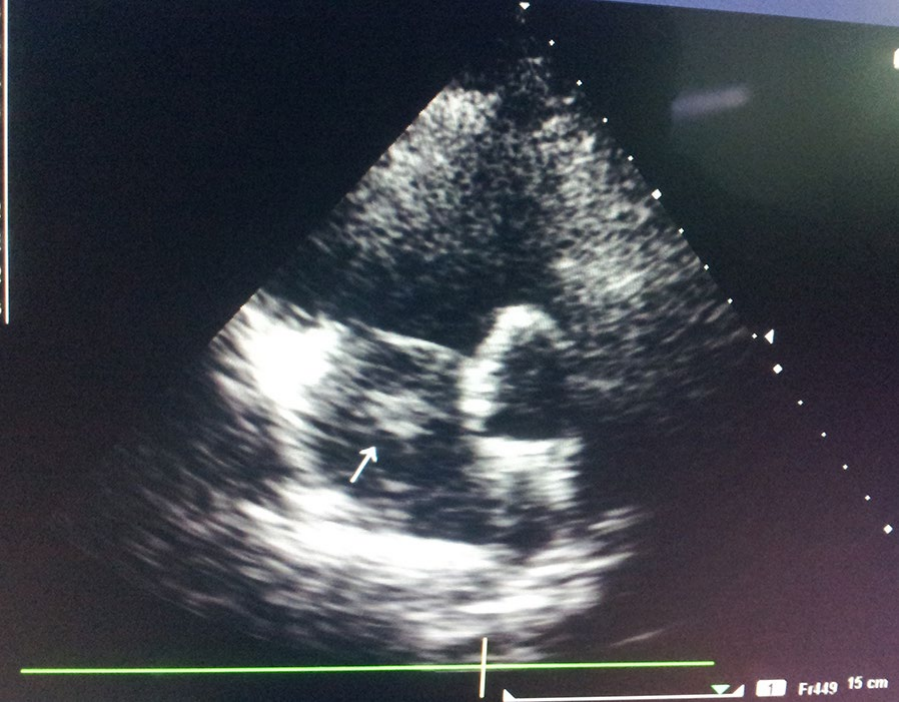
Trans-thoracic echocardiogram showing thrombus. Two-dimensional echocardiography in apical five chamber view showing thrombus (*white arrow*) in the dilated right atrium
